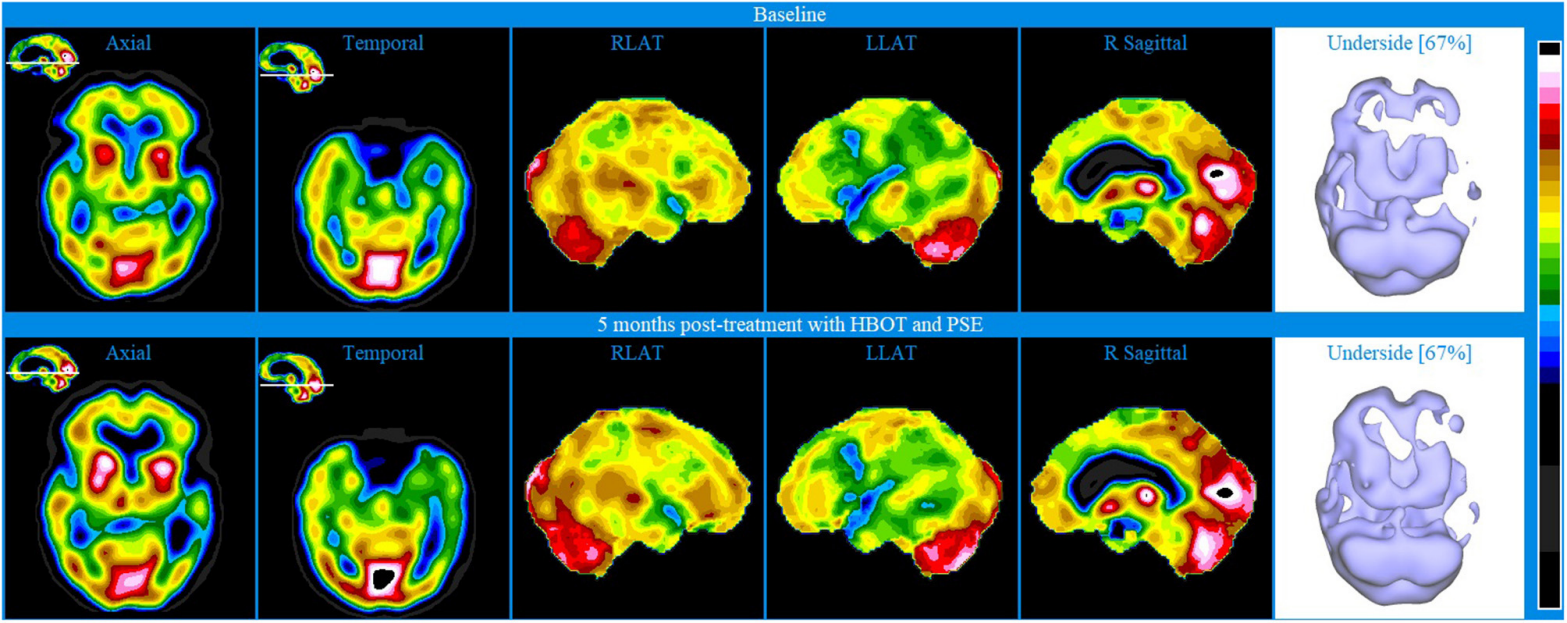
Brain SPECT images for Patient V. Top line relates to brain SPECT images at baseline. Bottom line shows SPECT images 5 months post-treatment with HBOT and PSE. Green arrows indicate areas of increased perfusion. In each row (from left to right) one axial slice and one temporal slice, three stereotactic surface projections of the right lateral (RLAT), left lateral (LLAT) and right sagittal and one iso-surface image of the underside.
